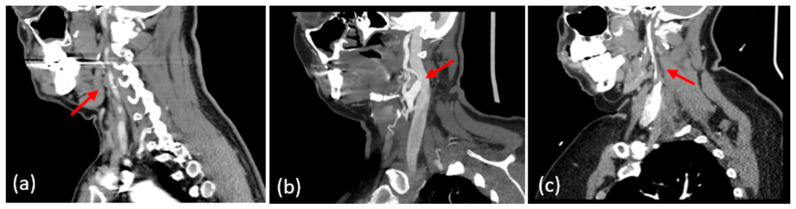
Representative CTA phenotypes. (a) Carotid atherosclerotic stenosis with focal luminal narrowing (red arrow). (b) Carotid artery dissection with tapered lumen and intimal flap (red arrow). (c) Fibromuscular dysplasia showing multifocal “string-of-beads” narrowing of the distal cervical ICA (red arrow).
